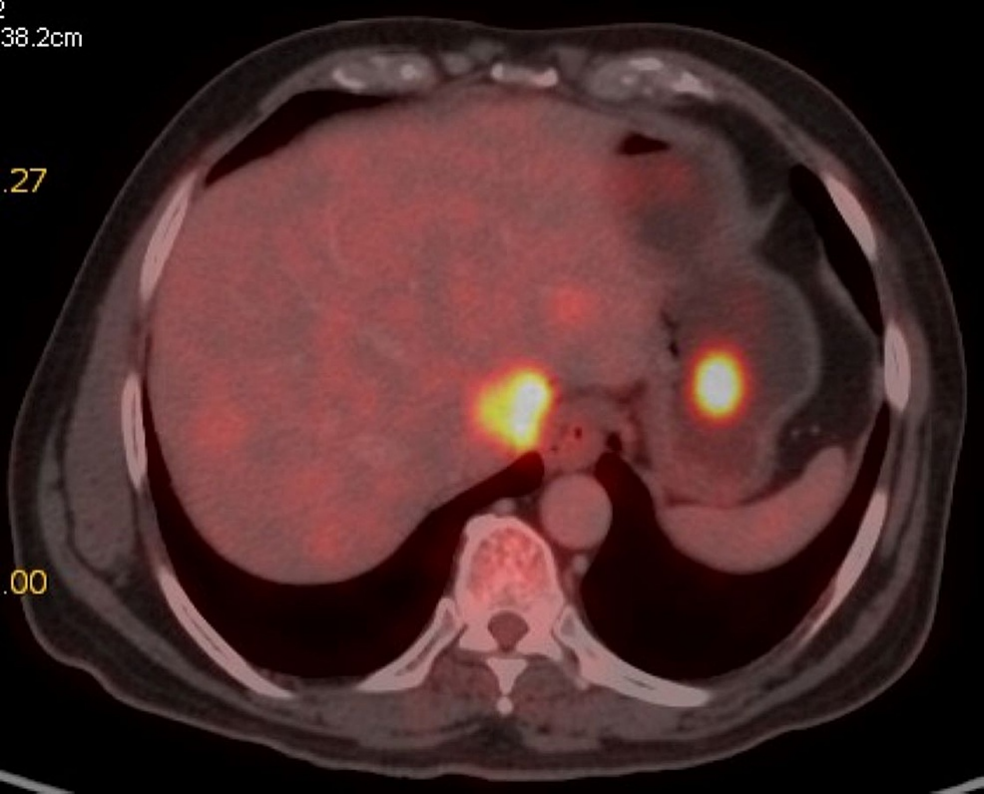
Baseline PET-CT scan showing lesions in liver (size 2.8 x 2.9 cm, SUVmax = 23.07) and stomach (size 1.9 x 2.1 cm, SUVmax = 13.05). PET-CT, positron emission tomography-computed tomography; FDG, fluorodeoxyglucose; SUVmax, maximum standardized uptake value.
